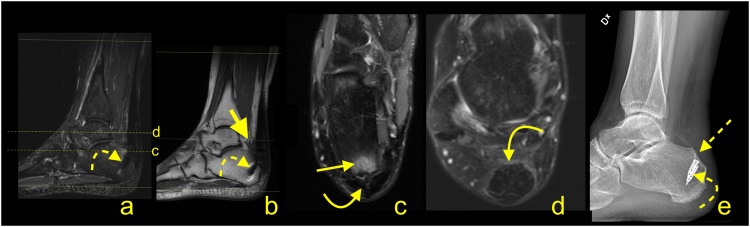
Normal status after the operation for Haglund's syndrome 9 months ago. Slight pain in the distal 1/3 of the Achilles tendon. MRI (a–d) and X-ray (e) revealed screws (curved dashed arrow) in the calcaneus and discrete postoperative bone marrow edema (straight arrow). The Achilles tendon is thick and fusiform but without rupture (curved arrow). Postoperative adhesions are visible in Kager's fat pad (thick arrow). A- T2- weighted with fat suppression, b- T1- weighted, c and d- PD- weighted with fat suppression, e- the lateral projection of the ankle joint.
